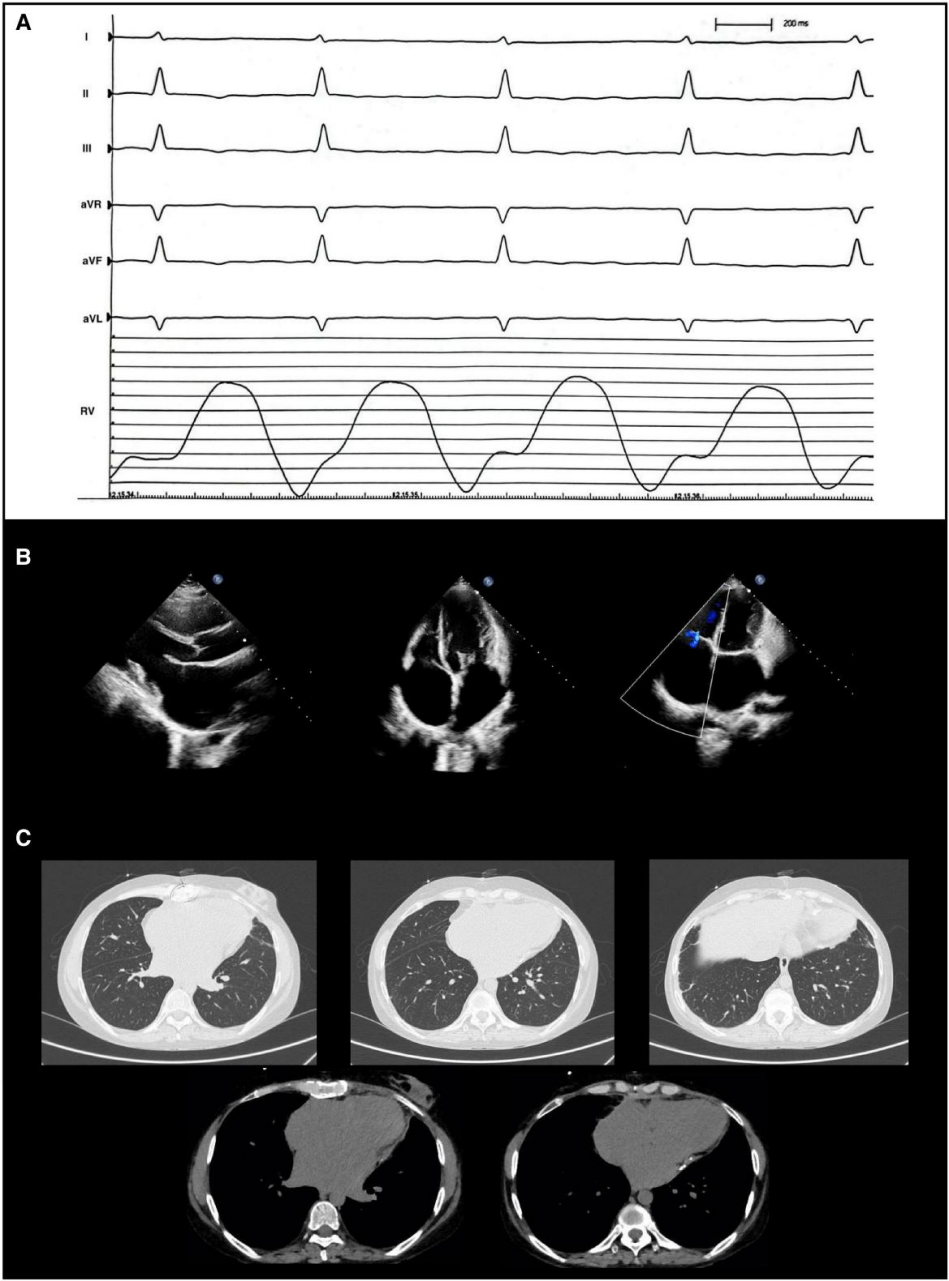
Therapeutic response to immunosuppression. Right heart catheterization (*A*), echocardiogram (*B*), and computed tomography thorax (*C*) after 18 months of mycophenolate immunosuppression. Note the normalization of right ventricular pressure curves, reduction in biatrial dilation, and mitral and tricuspid regurgitation; pulmonary window computed tomography scans demonstrate resolution of pulmonary nodules and pleural effusion (*C*, top), while mediastinal window computed tomography scans show reduction in pericardial calcifications (*C*, bottom) compared with post-pericardiectomy computed tomography scans.
